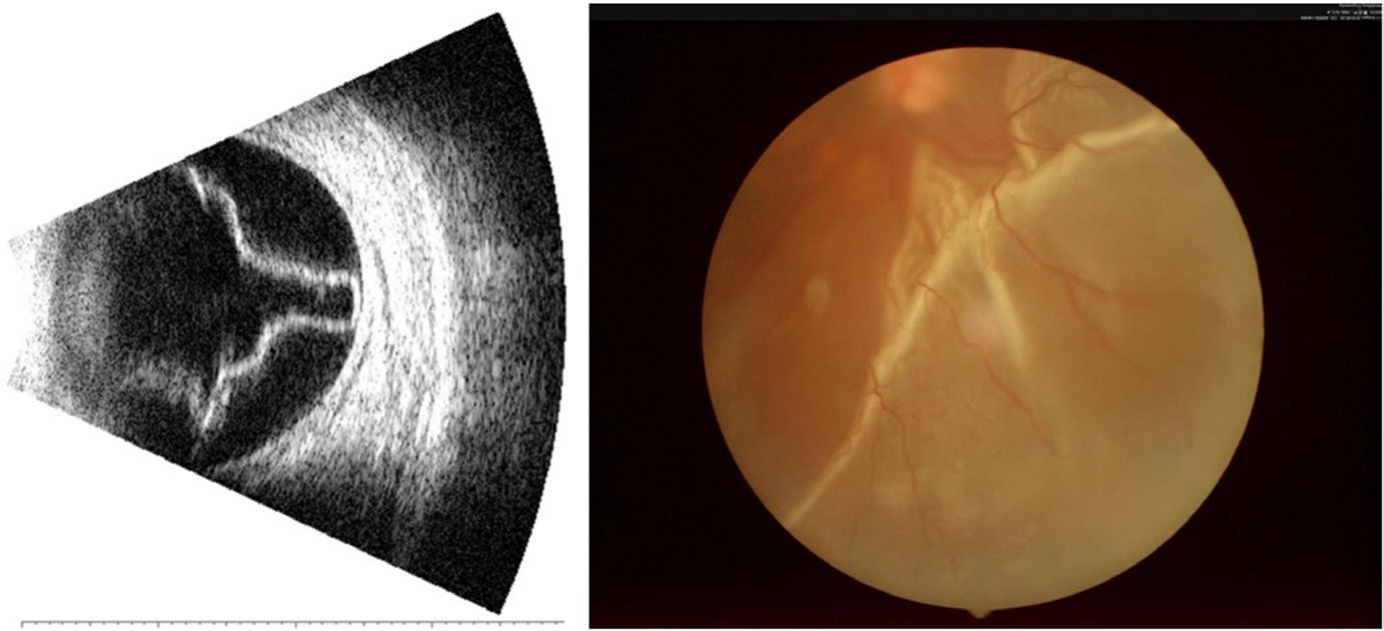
Example of RD seen on B‐ultrasound (left) and fundus (right)
